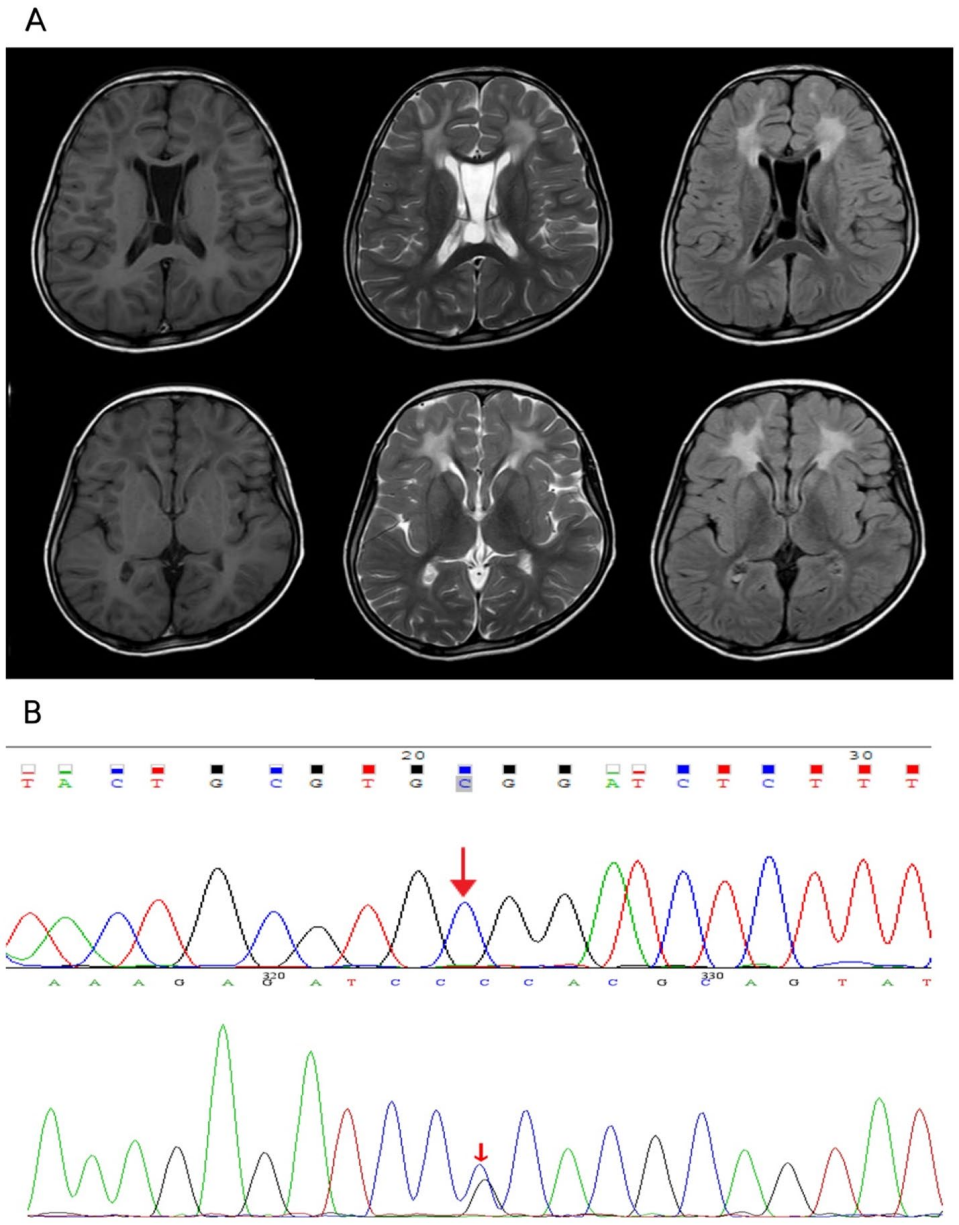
(A) Patchy slightly longer T1 and longer T2 signals were seen in the white matter of bilateral frontal lobe, bilateral basal ganglia area and cerebellum, brainstem, FLAIR showed higher signal, with a more symmetrical distribution bilaterally, corpus callosum was thin, part of the sulcus of bilateral cerebellar hemispheres was deep and wide, the interspace between the septum pellucidum was wider, the widest part was about 19.7mm, the fourth ventricle was wider, the occipital pools were larger, and no displacement of the midline structure was seen. A few T2WI high signal shadows were seen in the sinuses. (B) The patient had a monoallelic variant of the GFAP gene, while her father had no variation at this locus.
